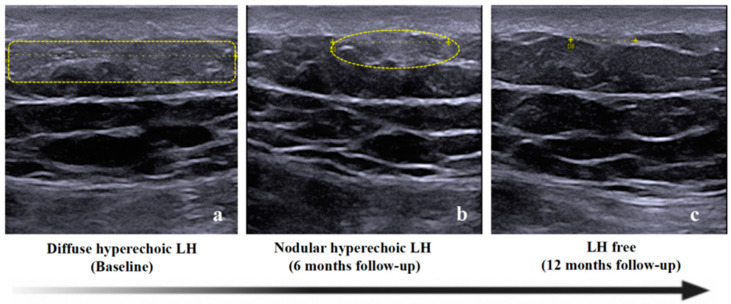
Examples of the changes in hyperechoic LH under ultrasound at follow-up. Diffuse hyperechoic LH at baseline (a) had obviously shrunk to nodular LH at 6 months follow-up (b). Additionally, the hyperechoic area could not be observed at 12 months follow-up (c) in the same patient (patient NO. 14).
